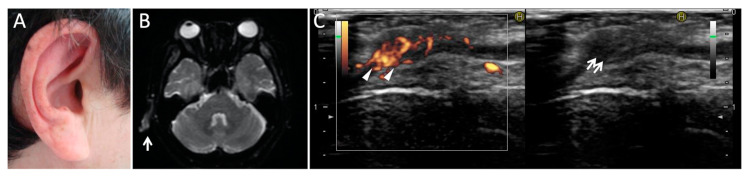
Magnetic resonance imaging and ultrasonography of auricular chondritis in a patient with optic perineuritis due to RPC. The right pinna is red and swollen (A) and diffusion-weighted magnetic resonance imaging (B) shows signal hyperintensity of the right auricle (arrow). Ultrasonography (C) reveals a high-power Doppler signal surrounding the cartilage (arrowheads) and swollen auricular cartilage (arrows).
